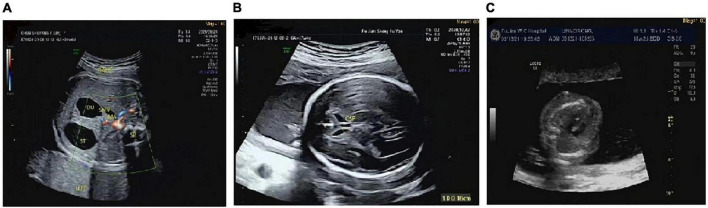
The prenatal ultrasound phenotype of the fetuses. (A) Showed duodenal obstruction. (B) Showed mild ventriculomegaly, dysplasia of the septum pellucidum, agenesis of the corpus callosum. (C) Showed a strong echo in the left ventricle.
